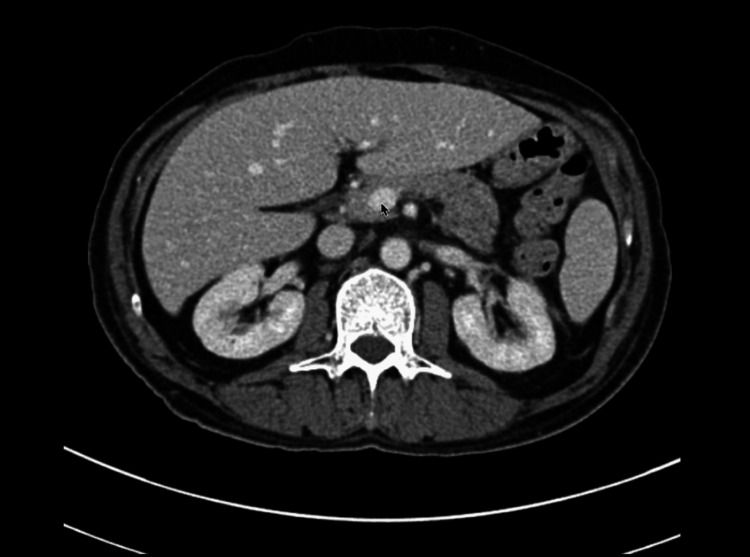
Axial contrast-enhanced CT abdomen showing no hepatosplenomegaly or lymphadenopathy
